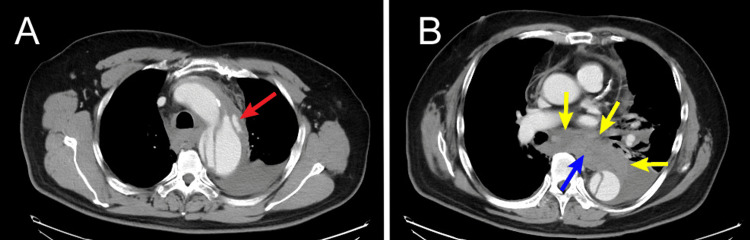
Preoperative contrast-enhanced computed tomography (A) Preoperative CECT showing a ruptured dissecting aneurysm of the distal aortic arch. The rupture site originates from the false lumen along the greater curvature, with contrast extravasation clearly visualized (red arrow). (B) Preoperative CECT demonstrating a hematoma(yellow arrow) surrounding the esophagus(blue arrow), consistent with mediastinal extension of the rupture.
